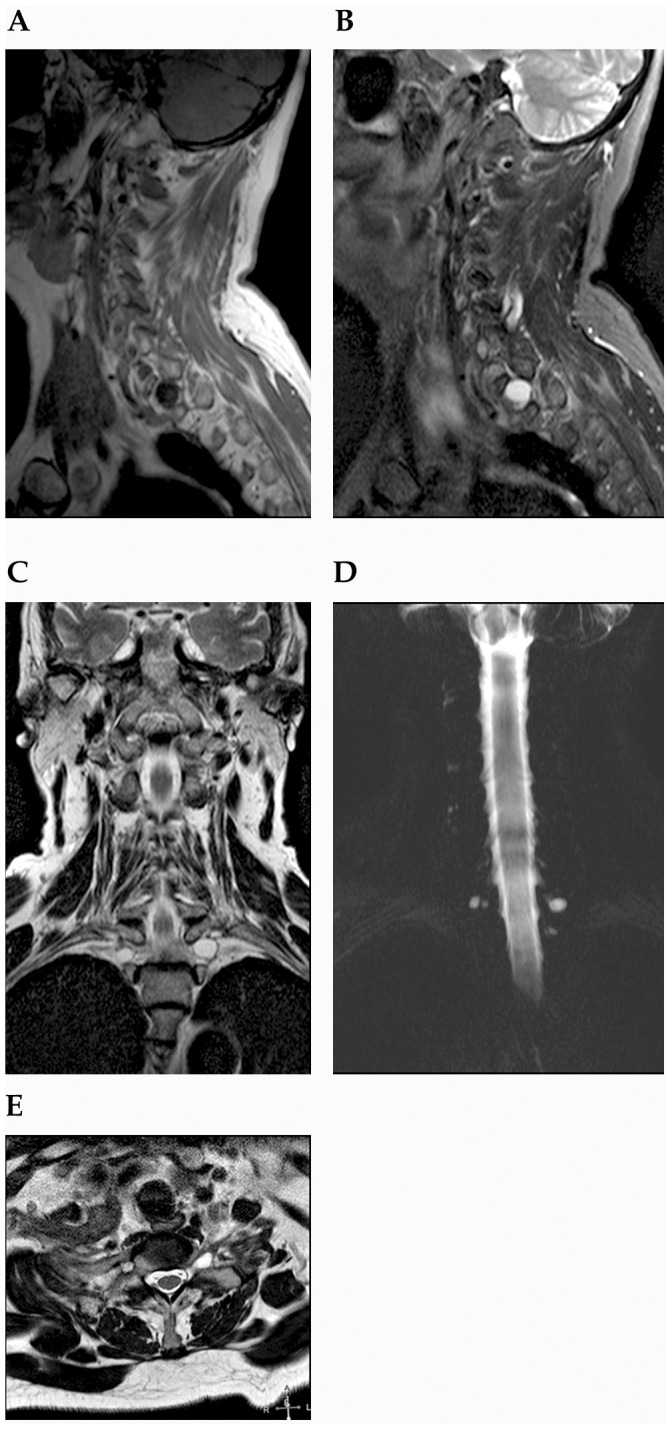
63-year-old woman with bilateral perineurial cysts on the T1 level (between T1 and T2). Sagittal section on T1- (A) and FST2-weighted (B) images with a view of the left lesion. Frontal T2-weighted section (C) and MR mielography (D). Axial T2-weighted (E) image.
